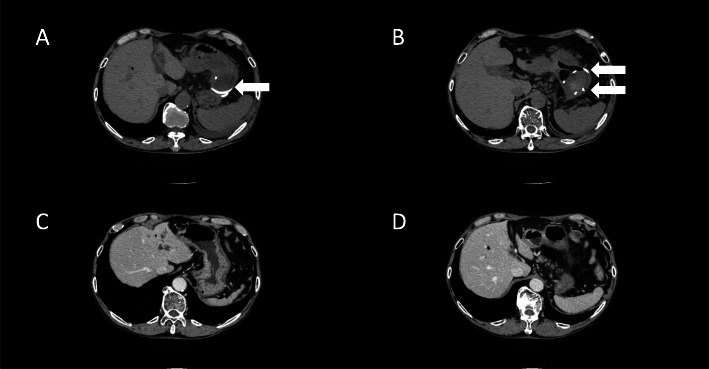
Computed tomography (CT) findings. a and b Plain CT scans taken on hospital day 26. A 5 Fr pigtail catheter was placed in the gastric submucosal abscess (arrows). c and d Contrast-enhanced CT scans taken 4 months after discharge. No gastric submucosal abscess and intra-abdominal abscess were shown
